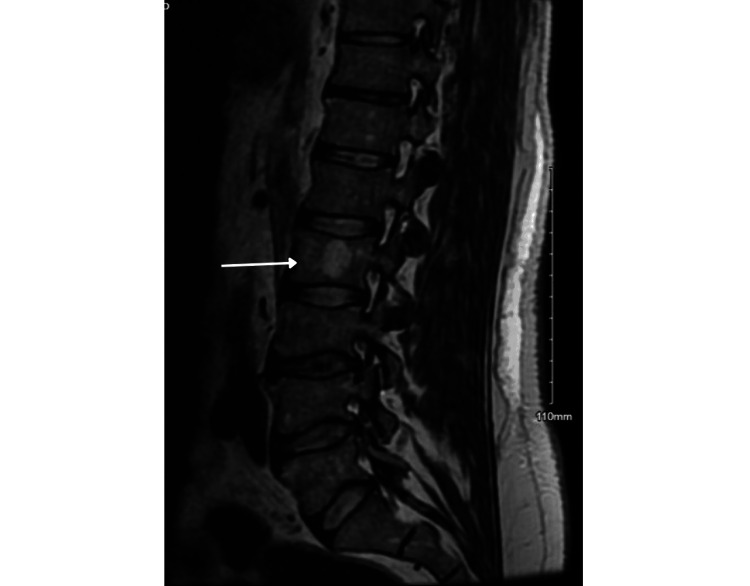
MRI lumbar spine demonstrating T1 and T2 intense lesions in the L2 likely representing intraosseous hemangioma.
